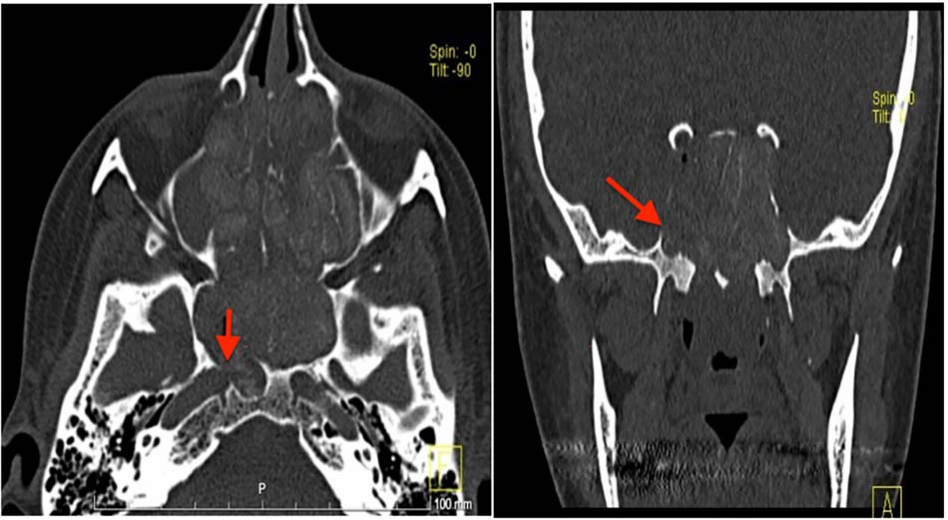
CT scan, left imaging is an axial view, without contrast, showing complete opacification of all paranasal sinuses with heterogeneous density inside the sinuses; with erosion in the distal part of the right petrous carotid canal abutting the right sphenoid sinus (arrow); right image is a coronal view, showing extensive polyposis with bony erosion in the bony boundaries of the sphenoid sinuses.
